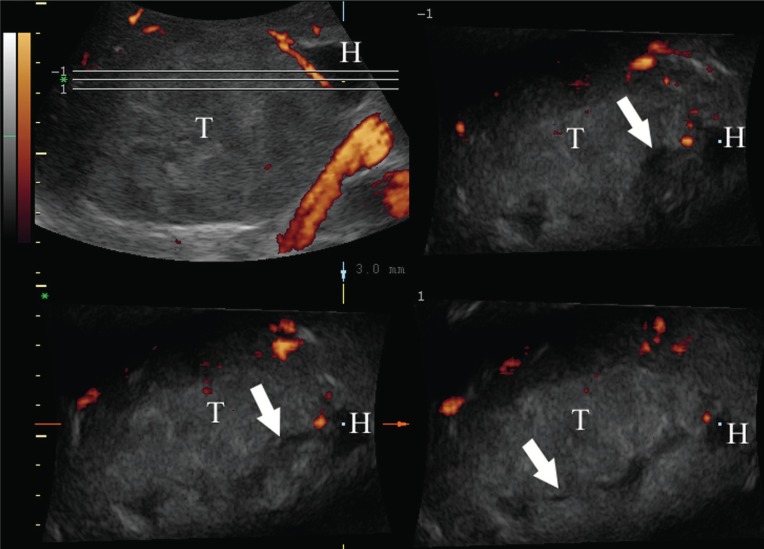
Three-dimensional sonography with power Doppler of the right adrenal carcinoma (T). Tomographic ultrasound imaging – TUI – is a postprocessing option enabling presentation of volume data on consecutive slices like with CT. On coronal pilot image (upper left) the position of consecutive slices in plane parallel to the ultrasound probe is marked. The extraadrenal haemorrage (H) is continuous with hipoechoic intratumoral haemorrage (arrows) seen in plane parallel to the ultrasound probe, which is unique for 3D sonography.
